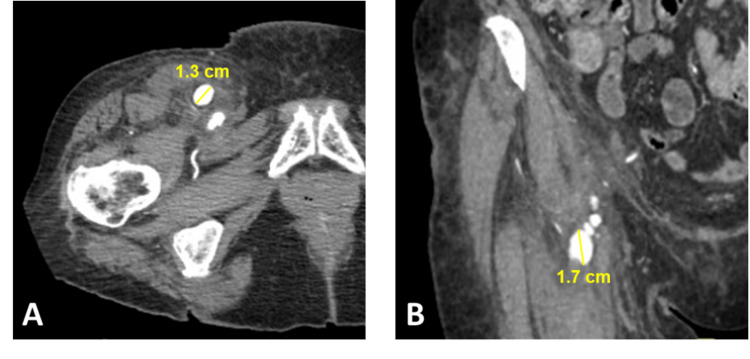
CTA abdomen/pelvis. The day following the initial interventional procedure, CTA was obtained demonstrating symptomatic pseudoaneurysm arising from the right common femoral artery with multiple lobulations, greatest dimensions noted in axial (A) and coronal (B) views. Adjacent soft-tissue edema noted. CTA, computed tomography angiography.
